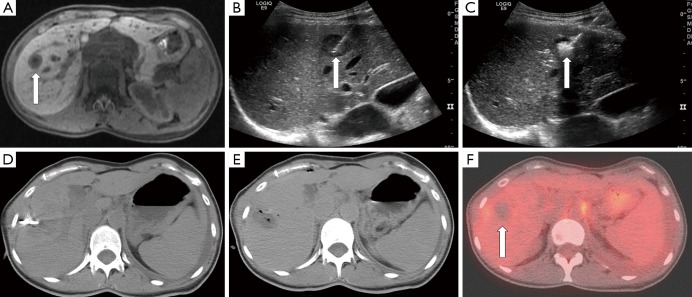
A 40-year-old female with metastatic breast carcinoma who presents with a focal metastatic tumor to segment 7 of the liver. (A) Axial post-contrast MRI image demonstrates 1.7×1.1 cm^2^ hypointense mass in segment 7 (arrow). Intraprocedural ultrasound images (B) During needle placement (arrow), (C) During ablation. Note the hyperechoic area which represents microbubble formation during heating (arrow). (D) Non-contrast axial CT image during procedure demonstrates applicator in place. (E) Non-contrast axial CT image immediately after ablation shows a hypodense region with focal air bubbles indicative of the ablation zone. (F) Fused axial PET-CT image 3 months post-ablation demonstrating ablation cavity with no evidence of residual FDG-avidity (arrow).
